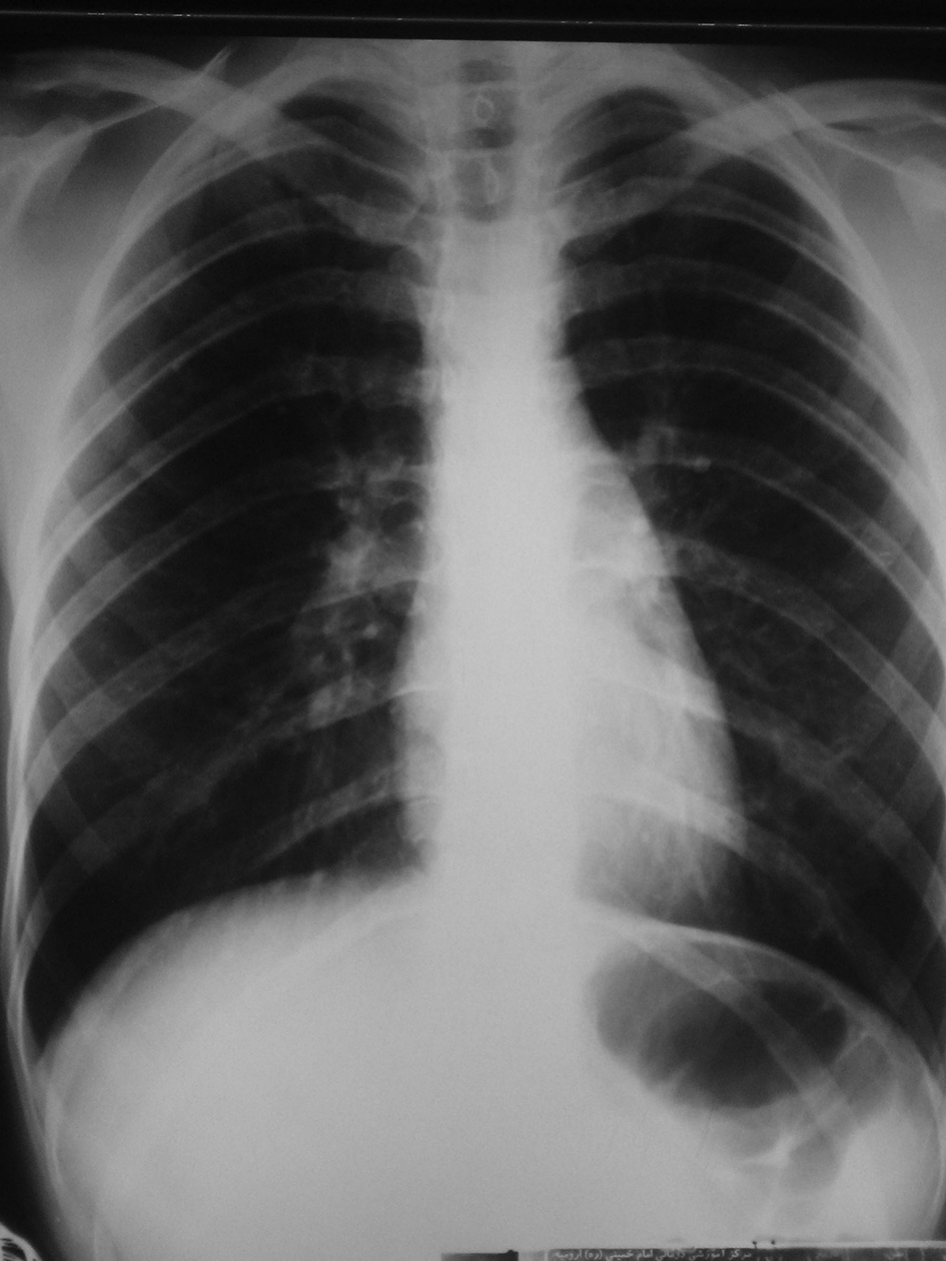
Posteroanterior chest radiograph shortly after treatment shows improvement of abnormalities
